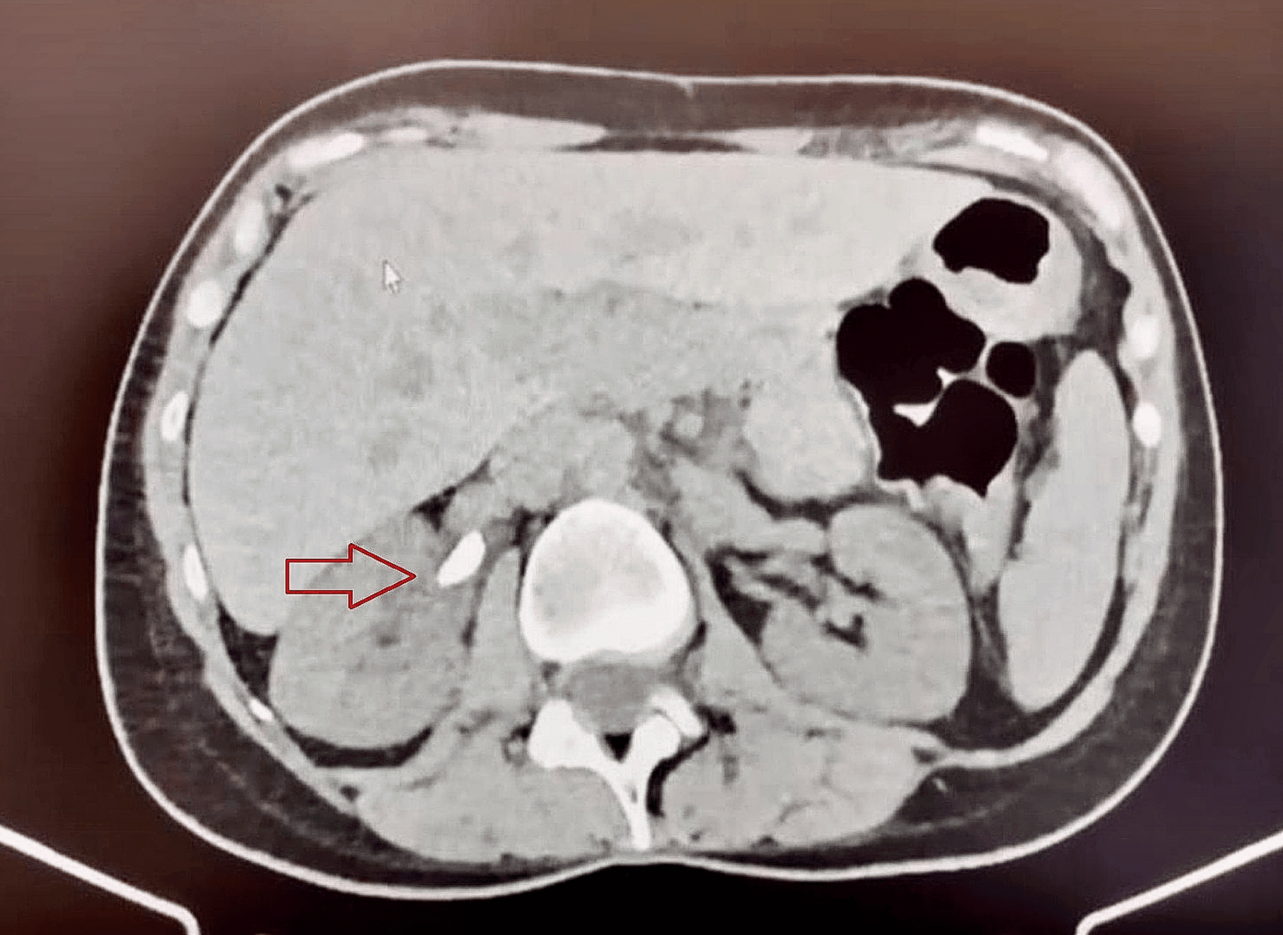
Preoperative computed tomography scan showing a 1.5 cm spindle-shaped urinary stone in renal pelvis
